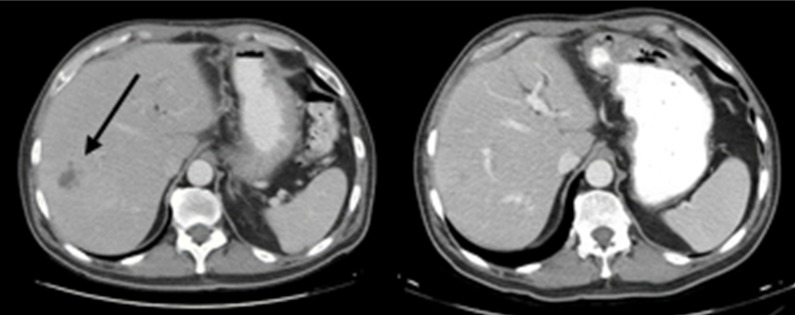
Axial CT images showing response of presumed liver metastases to chemotherapy The largest low attenuation liver lesion at the time of diagnosis (left with arrow), and repeat imaging 3.5 months later after receiving gemcitabine and oxaliplatin (right) showing interval resolution
